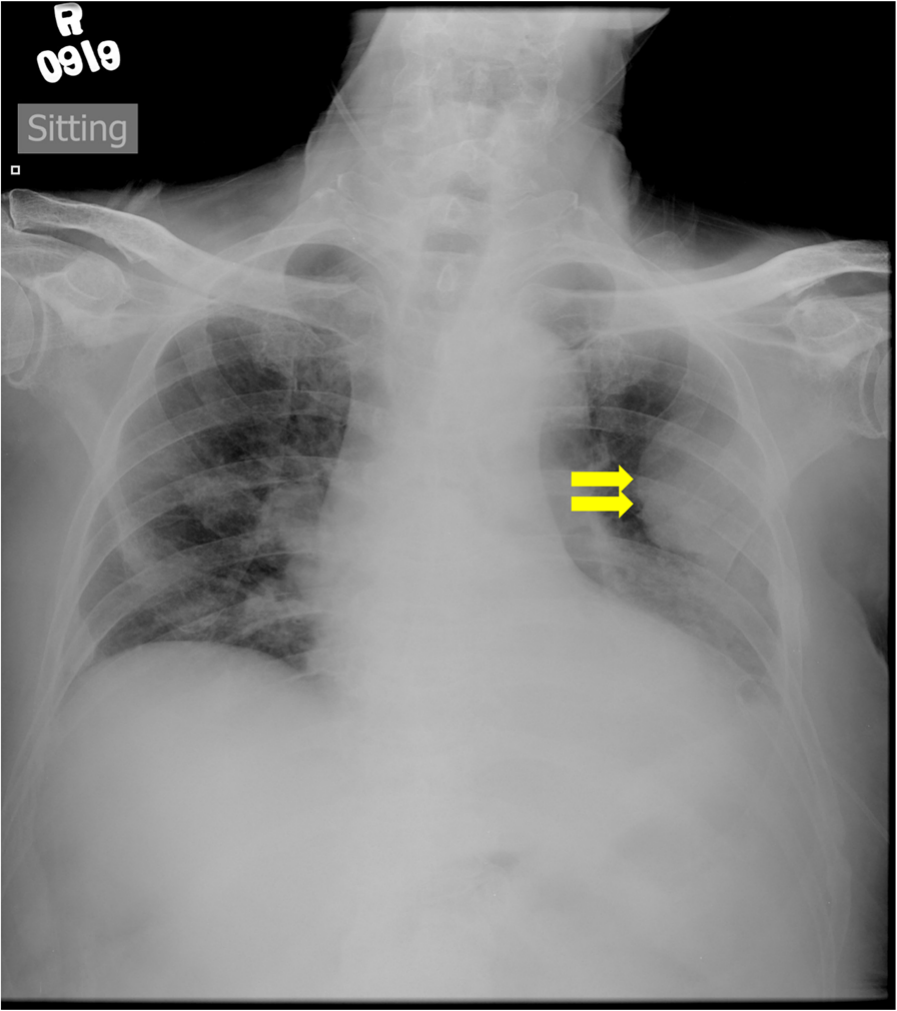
Radiographic image of the myeloma patient. Chest X-ray showed pleural masses after drainage of the myelomatous pleural effusion (arrows).
